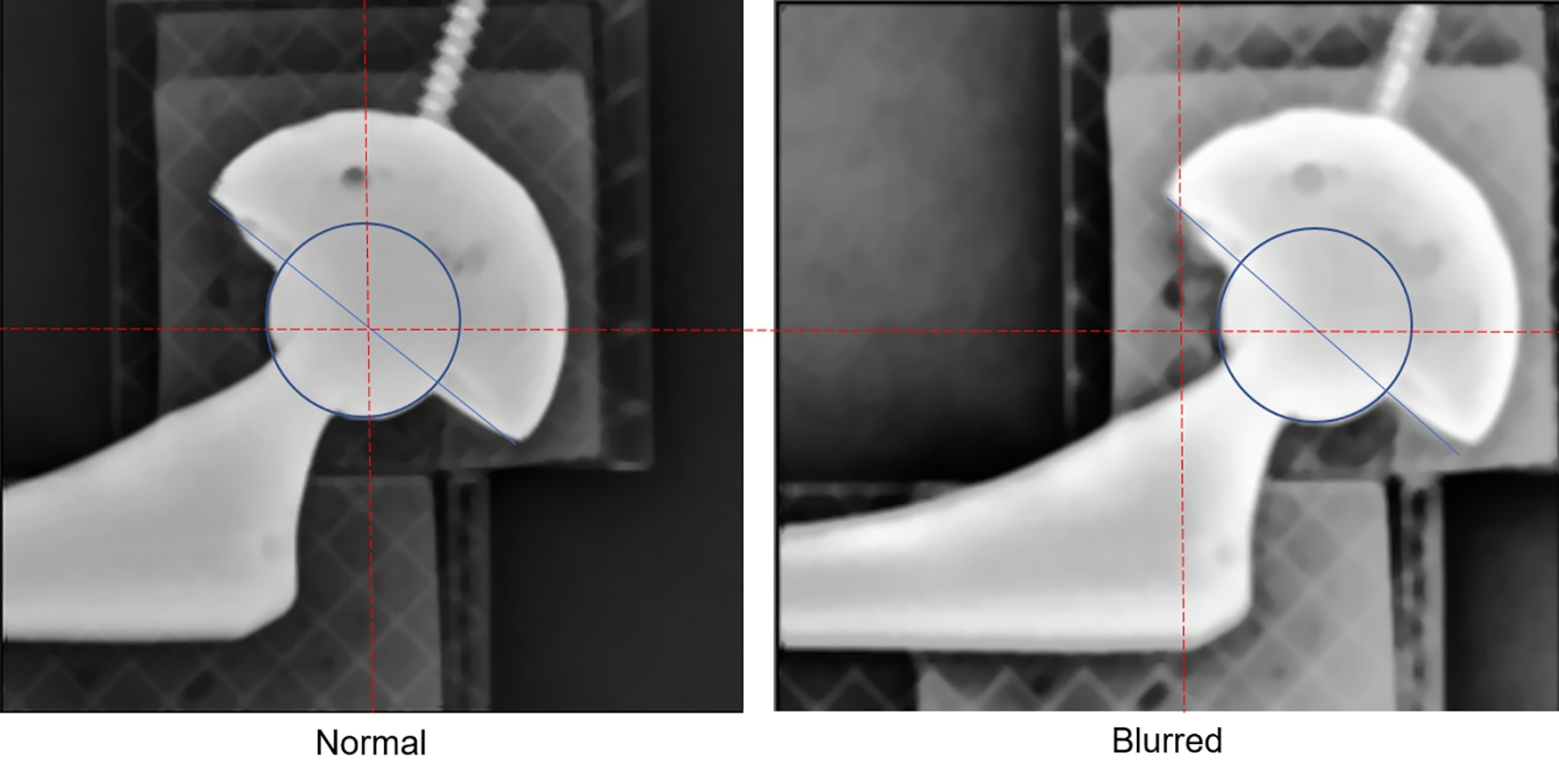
The blur of the edge detection area. For the same x-ray image, different specifications of rectangular edge detection areas result in different image sharpness. The left one is normal, but the right one is blurred. In the normal case, the rectangular edge detection area is specified such that its center is at the very center of the femoral head. In the blurred case the rectangular edge detection area is specified so that its center is considerably off the center of the femoral head, causing the edge detection area to blur.
